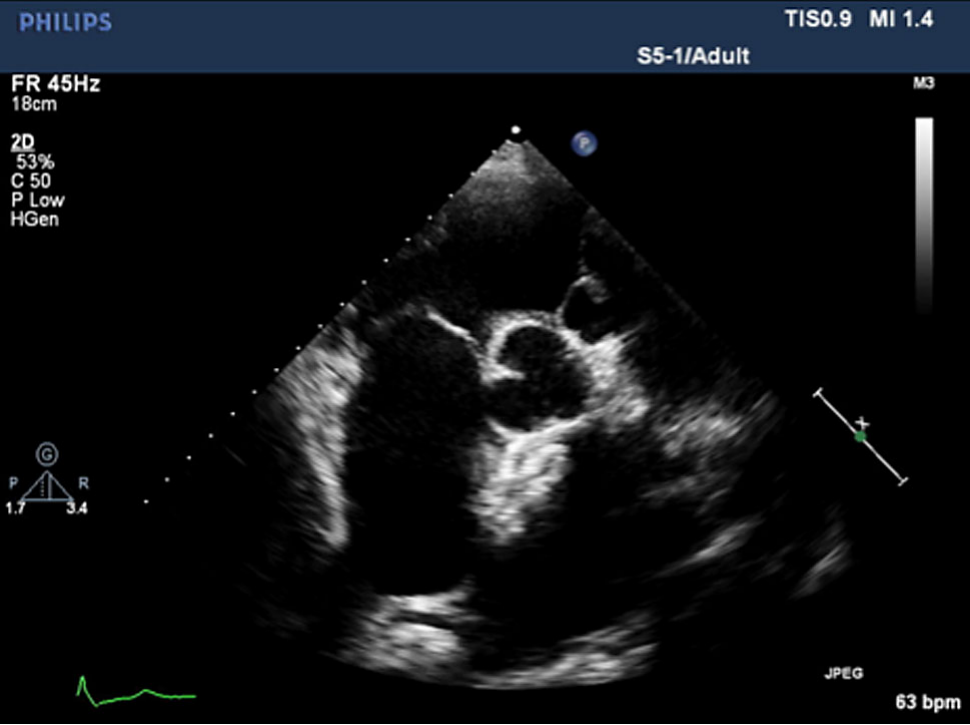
Transthoracic echocardiographic short axis view after 6 months of follow-up. The device contacts the sinus of Valsalva smoothly, and the pericardial effusion has not increased
